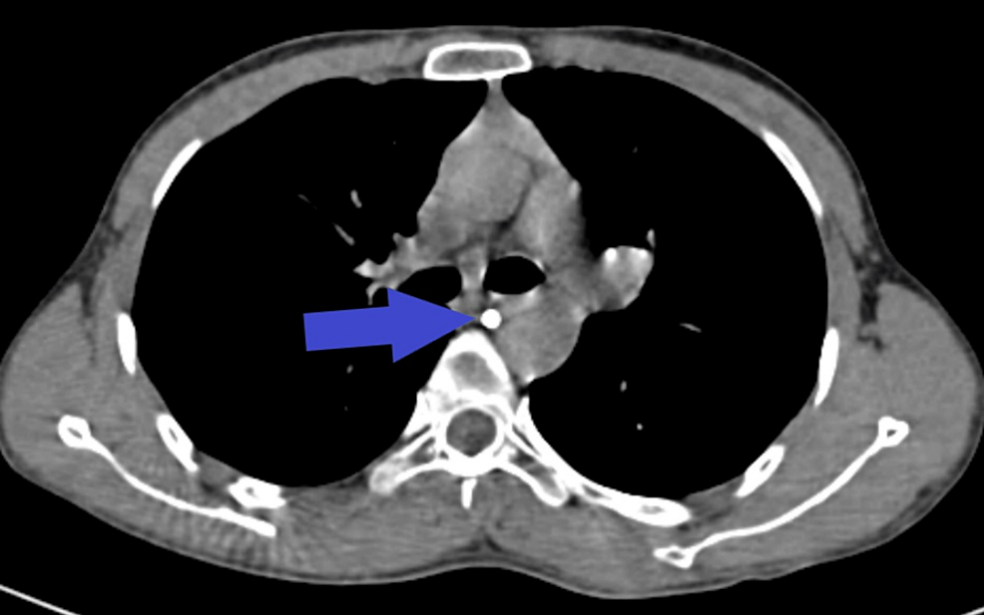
Post-embolization plain CT chest showing hyperdense material (arrow) in the main thoracic duct, suggestive of NBCA glue. NBCA: N-Butyl Cyanoacrylate.
